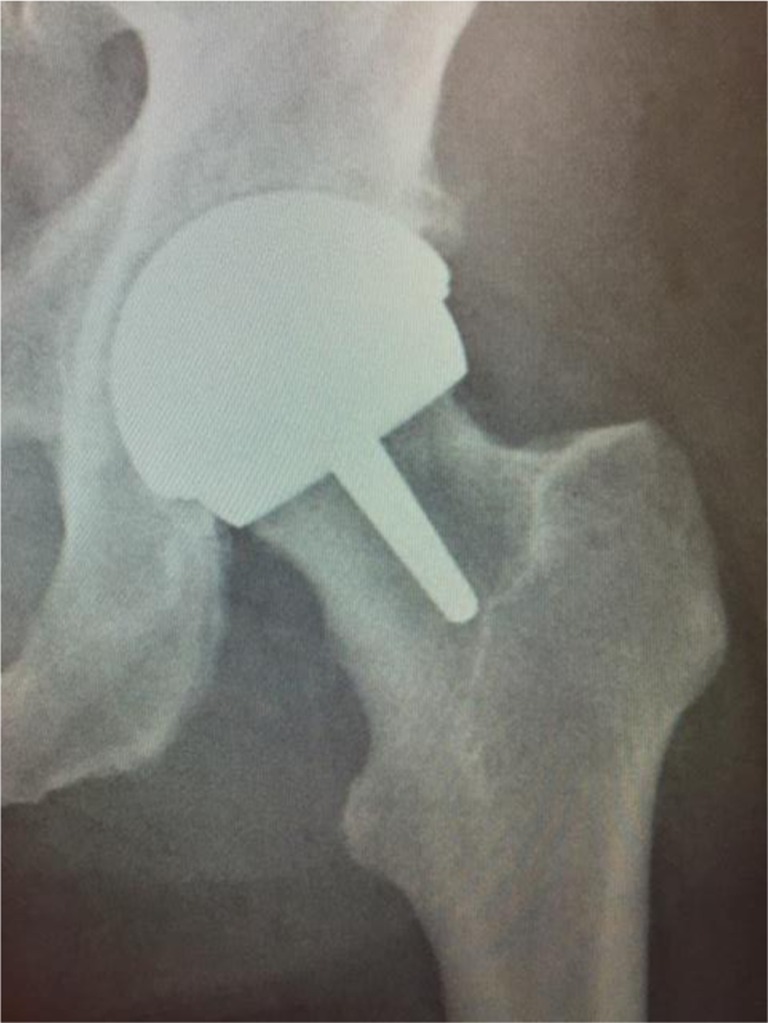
Post-operative anterior–posterior plain film radiograph of the patient’s left hip. No cause of the patient’s mechanical symptoms was detected
